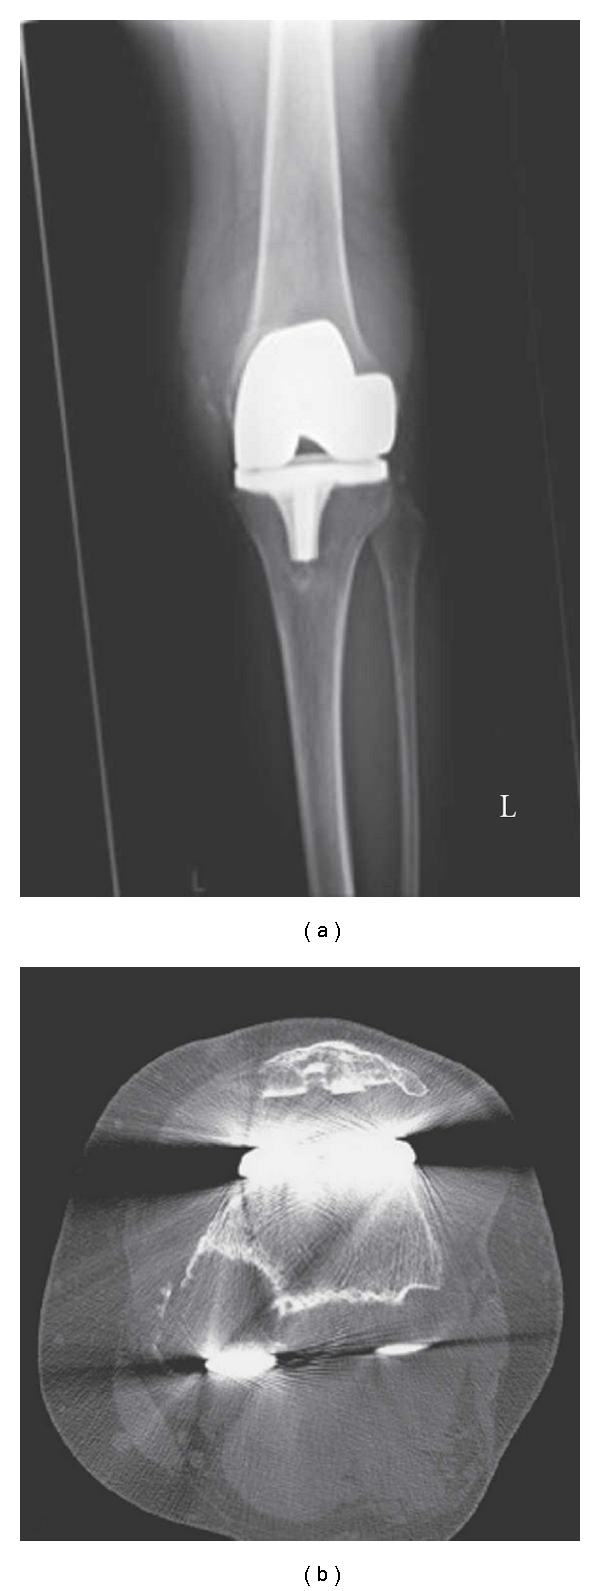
AP radiograph showing a knee with severe polyethylene wear and evidence of major bone loss (a). A CT scan showing massive bone loss of the medial and lateral femoral condyles due to osteolysis (b). (reprinted from Backstein et al. [2]).
